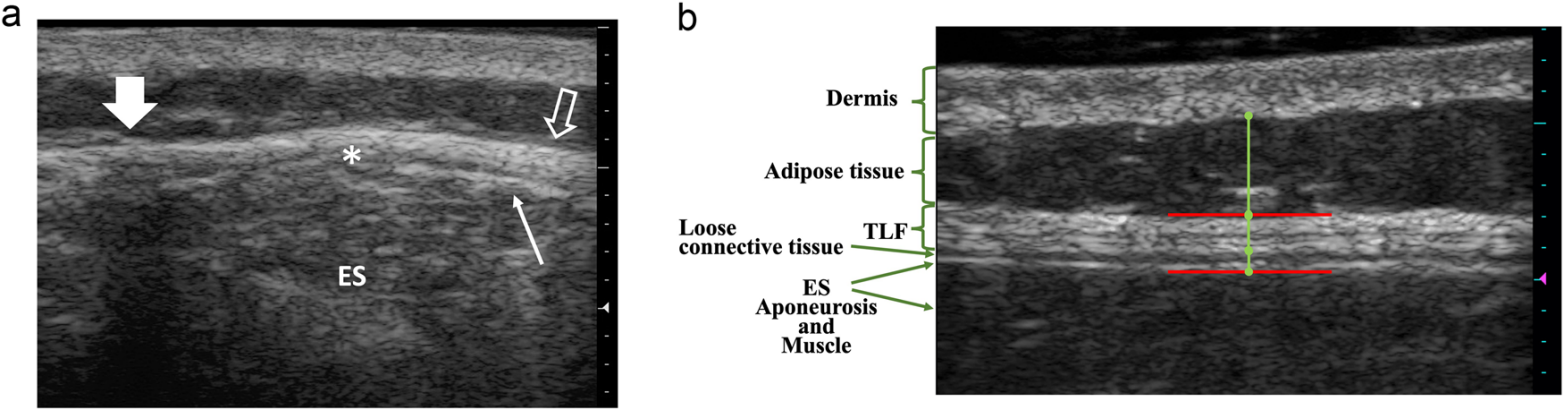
Ultrasound imaging and layer identification of paraspinal soft tissues. a Transverse (short-axis) and (b) longitudinal (long-axis) B-mode images of the right paraspinal soft tissues in a 50-year-old man with NSLBP. a The transverse image at the L2–L3 interspinous space (large arrow) shows the ES muscles with their hyperechoic aponeurosis (thin arrow) and the overlying TLF (open arrow). The peak convexity of the ES muscles (asterisk) was marked on the skin, and scanning was performed at this marked position in the longitudinal plane. b The longitudinal image displays the paraspinal tissues’ ultrasound anatomy, including the dermis, subcutaneous adipose tissue, multilaminar TLF, hypoechoic hyaluronan-rich loose connective tissue layer, hyperechoic ES aponeurosis, and muscles. For outlining paraspinal tissue layers, the medical students placed dots along the soft tissue layer margins on the image center’s first frame of the table-stationary phase. An algorithm then measured the thickness of the subcutaneous adipose tissue, TLF, and juxtamuscular zone (vertical green lines). The juxtamuscular zone included the hypoechoic hyaluronan-rich loose connective tissue and the hyperechoic ES aponeurosis. Distances were recorded in pixels and converted to millimeters using a 0.026 mm/pixel resolution. On the middle frame of the table-downward phase, two 10-mm red lines were positioned at the upper TLF and lower ES aponeurosis margins (shown together for convenience), defining the region of interest for ShS calculation. TLF, thoracolumbar fascia; NSLBP, nonspecific low back pain
